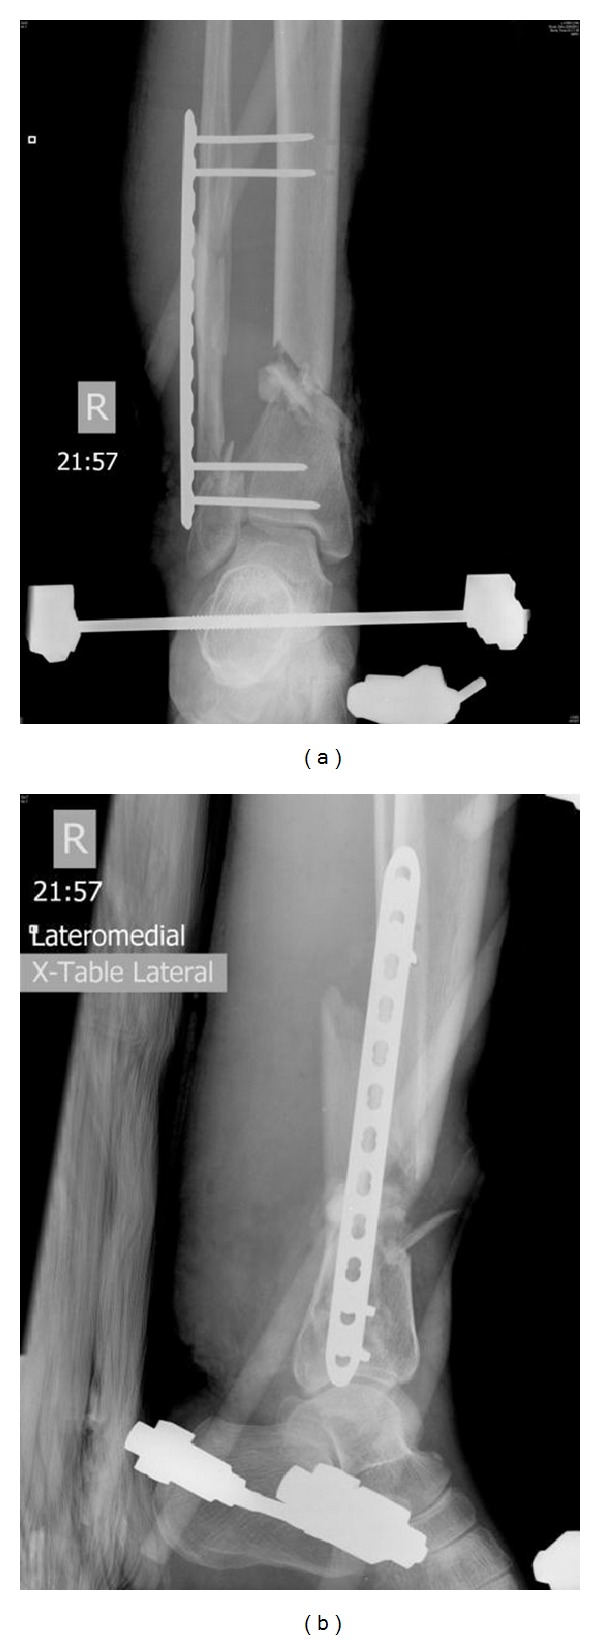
AP (a) and lateral (b) radiographs of an open fracture right distal tibia Gustilo Type IIIA at admission. It was initially debrided, stabilized, and shortened with an external fixator, leaving a defect over right distal tibia.
